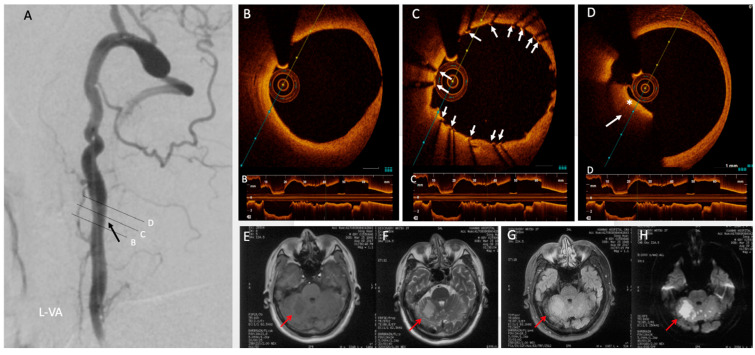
Post-stenting angiography and OCT images and post-procedural MRI. (A) DSA of the left V2 segment post-stenting showed marked improvement in previously severe stenosis, well-covered by the stent (black arrow). Cross-sectional OCT images during standard pullback demonstrating (B) normal lumen proximally with no thrombus and (C) satisfactory stent wall apposition (white arrow), whereas (D) distally a hyper-reflective area (*) projecting into the lumen with apparent posterior shadowing indicated red thrombus (white arrow) attached, which had no stent coverage on OCT, suggesting embolization risk. (E–H) MRI images following intervention when the patient developed clear symptoms demonstrate right cerebellar infarction (red arrow), presumed to be related to red thrombus, which was not covered.
